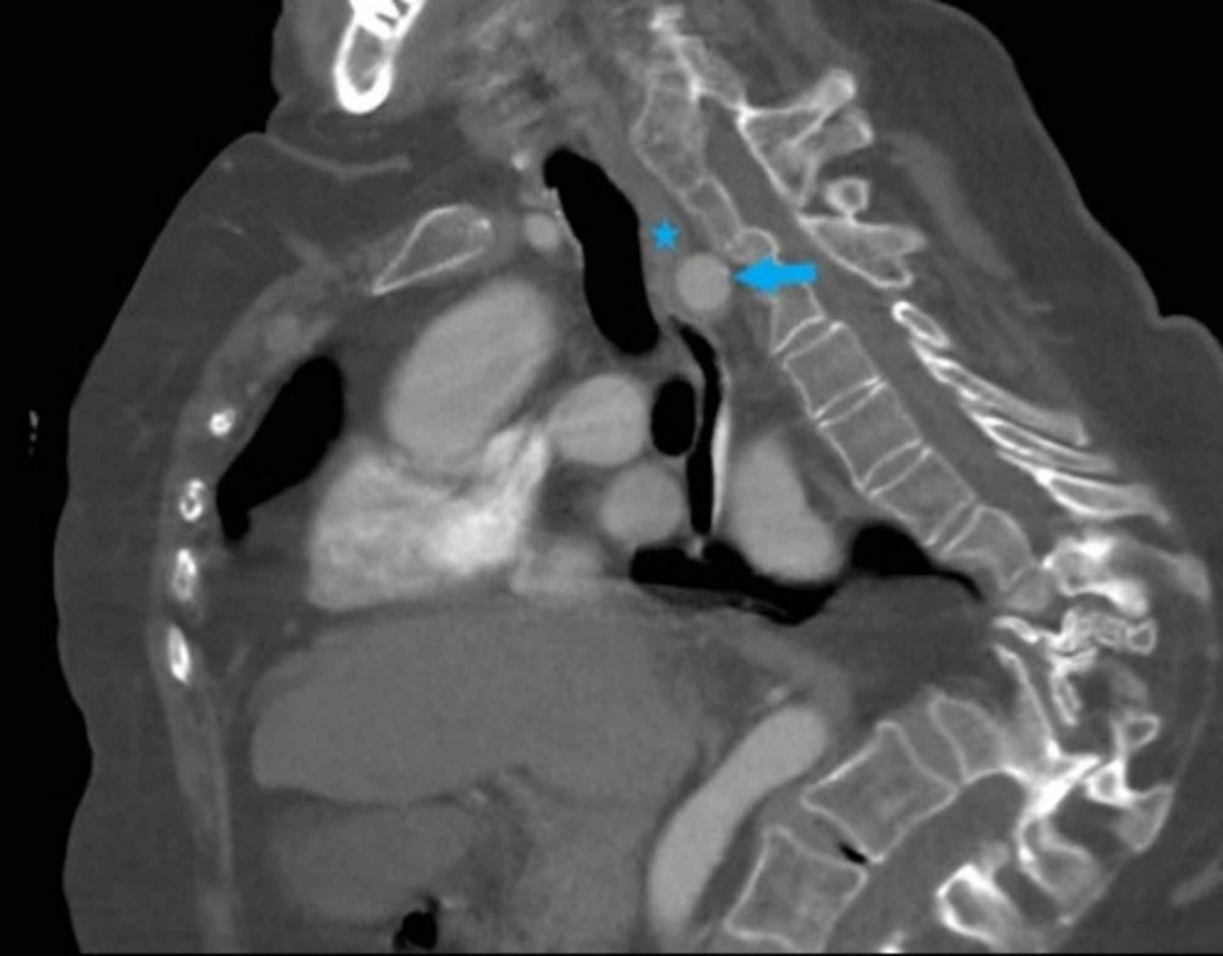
Chest contrast-enhanced computed tomography (CT) in the venous phase, sagittal view, showing an aberrant right subclavian artery passes behind the esophagus (asterisk) and trachea.
